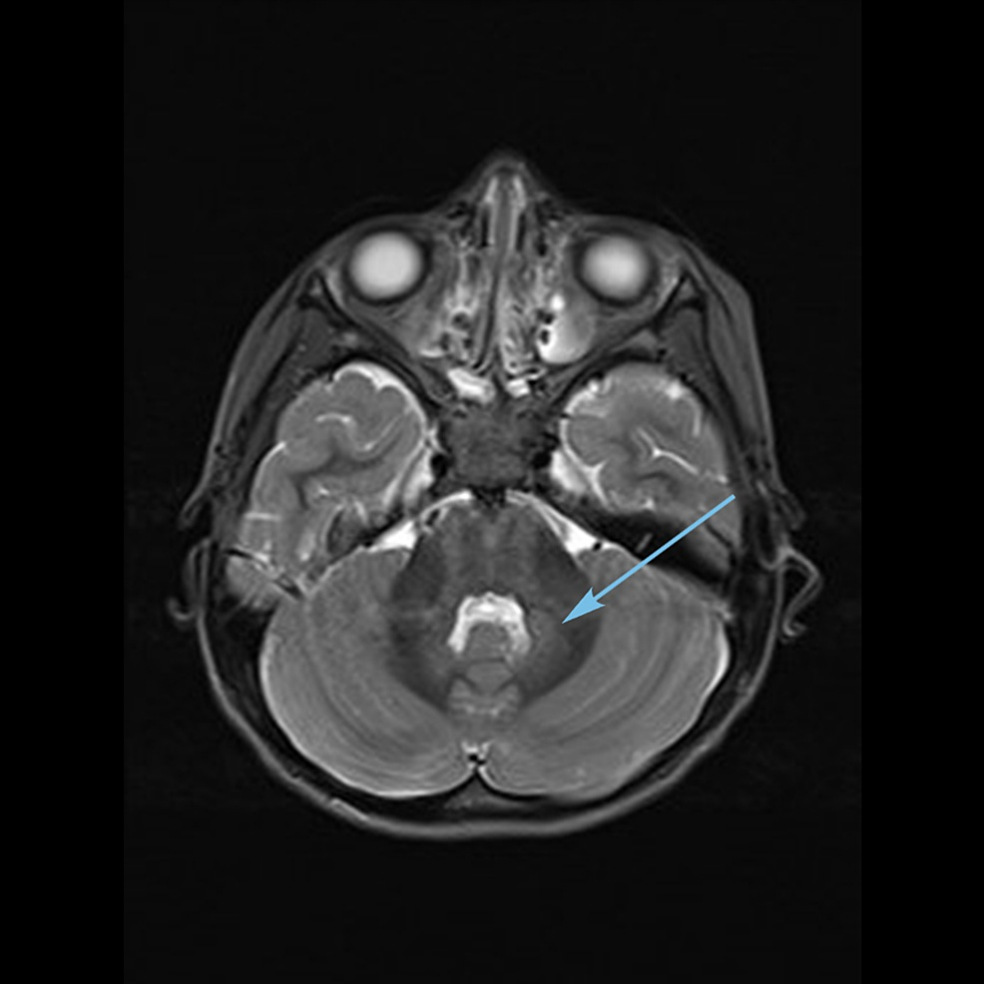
MRI brain T2 axial image showing dentate nuclei hyperintensities (arrow).
